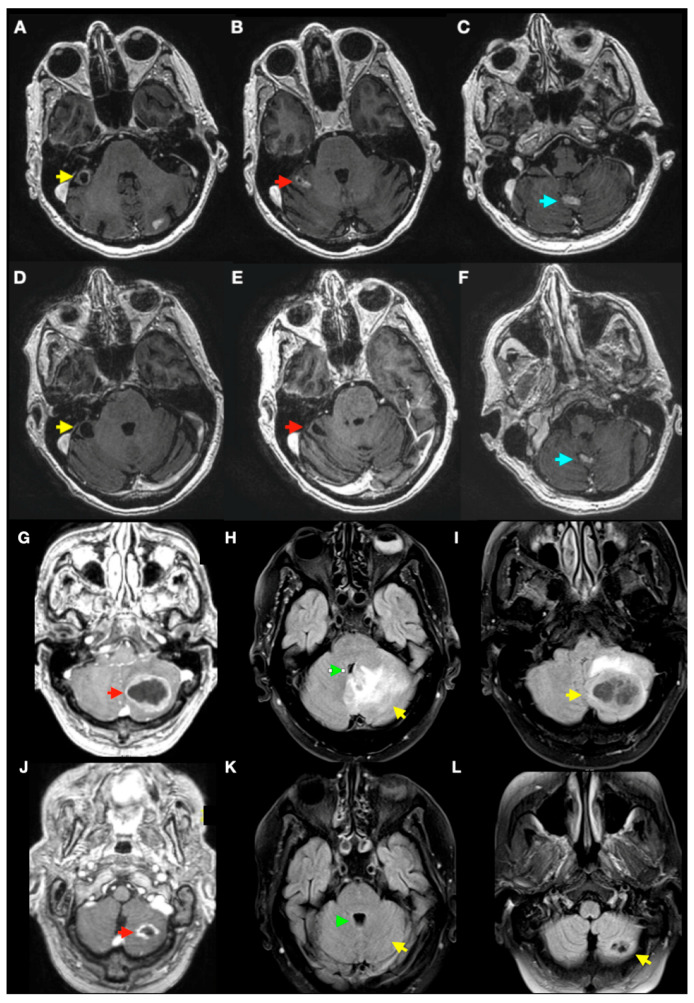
Cerebellar BMs MRI images before and after VMAT-SRS treatment. Axial 3D T1-weighted post-gadolinium images of posterior fossa brain metastases (PFBMs) before and after VMAT-SRS. For each case, the top row shows pre-treatment and the bottom row post-treatment. Case 1 (A–F): patient with multiple posterior fossa metastases. ((A,D), yellow arrows): flocculonodular lesion with cystic change and enhancing mural/irregular components pre-SRS (A); on follow-up (D), contrast enhancement has resolved, leaving a malacic cavity. ((B,E), red arrows): right cerebellar hemispheric metastasis with irregular enhancement pre-SRS (B); on control imaging (E), the enhancement has disappeared with a small residual malacic cavity. ((C,F), blue arrows): vermian metastasis pre-SRS (C) showing a partial response on follow-up (F) with reduction in size and enhancement. Case 2 (G–L): patient with a very large left cerebellar hemispheric metastasis causing marked fourth-ventricle collapse pre-SRS (G–I); post-SRS images ((J–L), two months later): significant tumour size reduction (red arrowheads; images (G,J)), marked reduction in oedema (yellow arrowheads; images (H–L)), and resolution of fourth-ventricle collapse (green arrowheads; images (H,K)).
